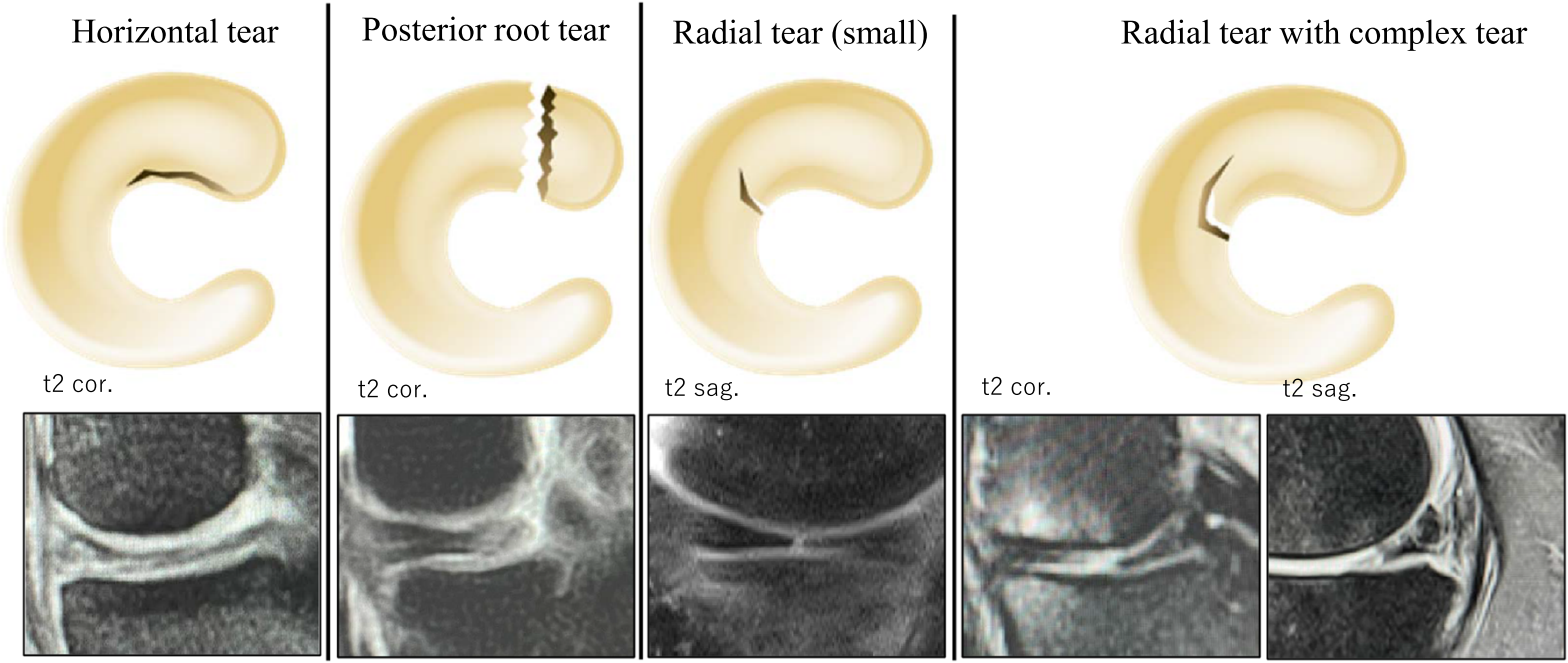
Magnetic resonance imaging assessment of meniscal tear type; magnetic resonance imaging. Cor; clonal. Sag, sagittal.
